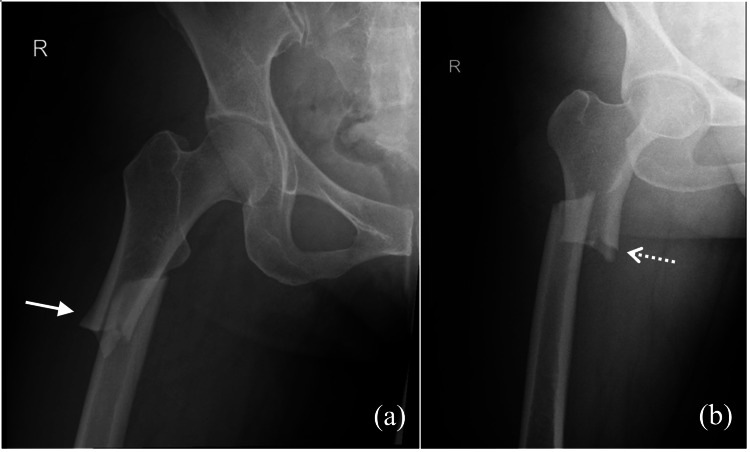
Radiographs of the right hip showing the transverse subtrochanteric fracture with lateral cortical thickening (solid arrow) and medial cortical spike (dotted arrow). (a) AP view and (b) lateral view
